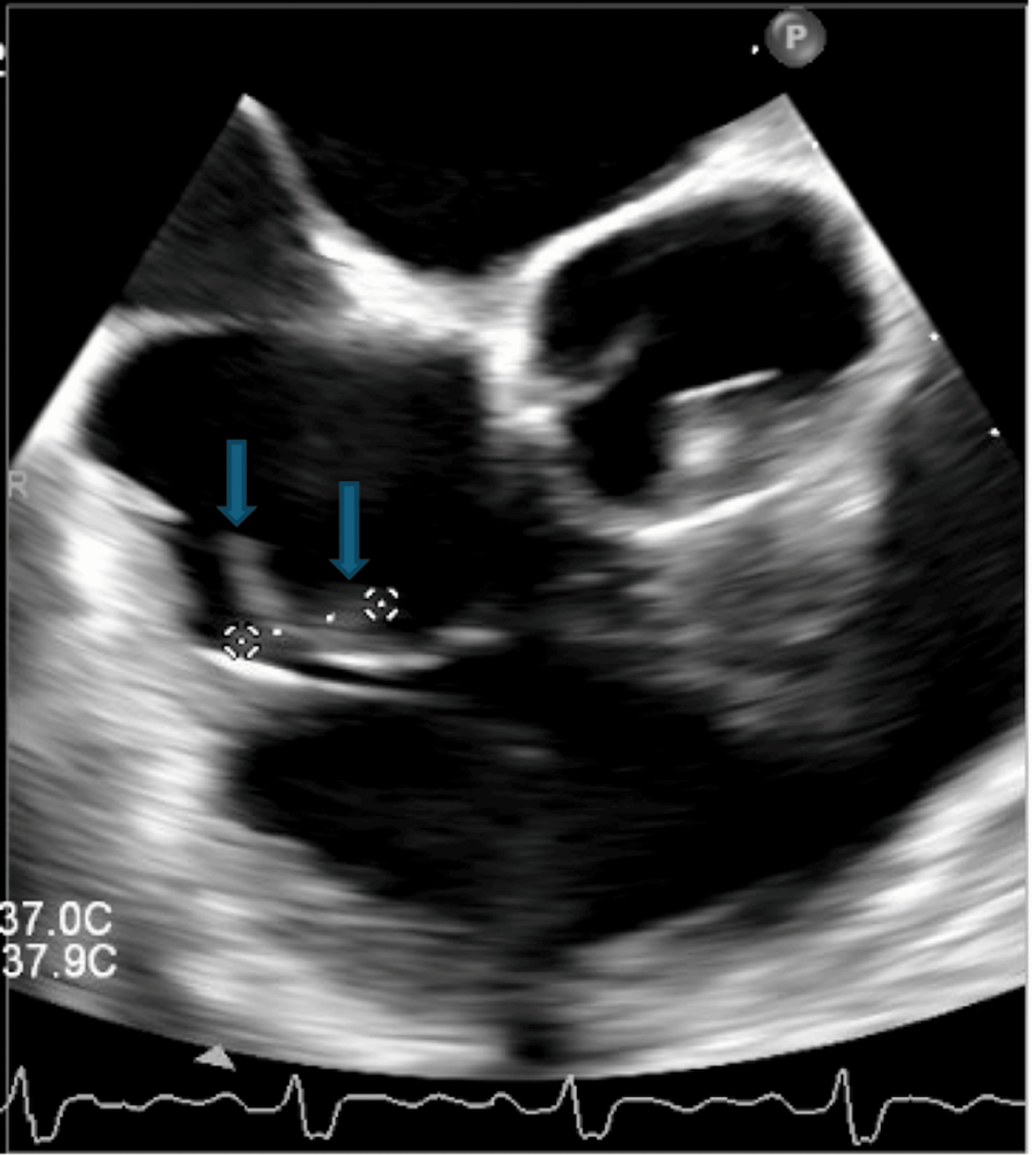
Transesophageal echocardiogram The image demonstrates a large vegetation measuring 1.32 x 1.35 cm adherent to the tricuspid valve (blue arrows).
